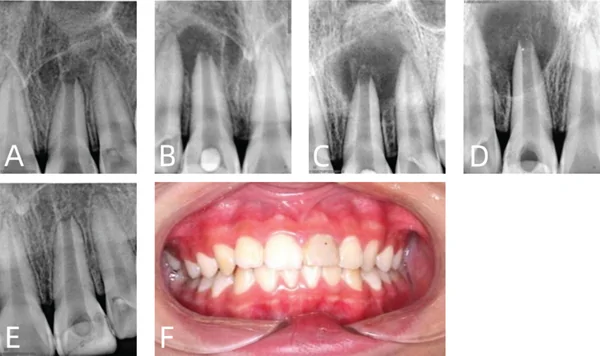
(A) Preoperative periapical radiograph; (B) second treatment visit; (C) 3‐month postoperative radiograph; (D) 12‐month postoperative radiograph; (E) 22‐month follow‐up periapical radiograph; and (F) intraoral photograph at the 22‐month follow‐up.
